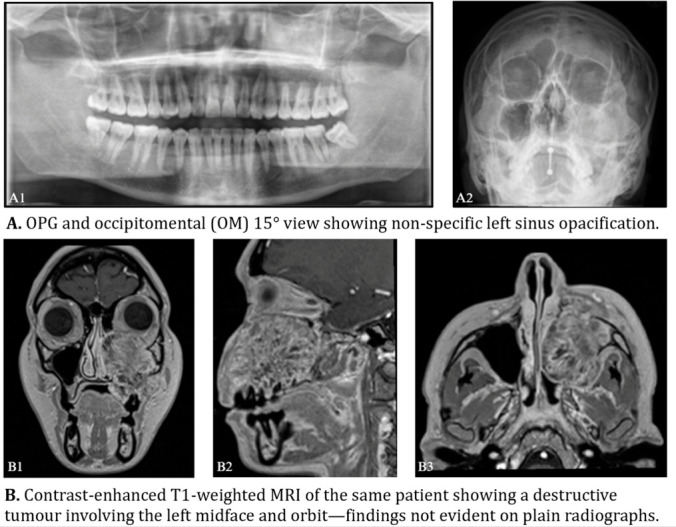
Comparison of plain radiography and cross-sectional imaging in a head and neck sarcoma. (A1,A2) Orthopantomogram and occipitomental radiographs showing left maxillary sinus opacification. (B1–B3) Contrast-enhanced T1-weighted MRI demonstrating a locally invasive soft tissue mass involving the left midface with extension into adjacent structures. Images reproduced with permission from NHS archives.
